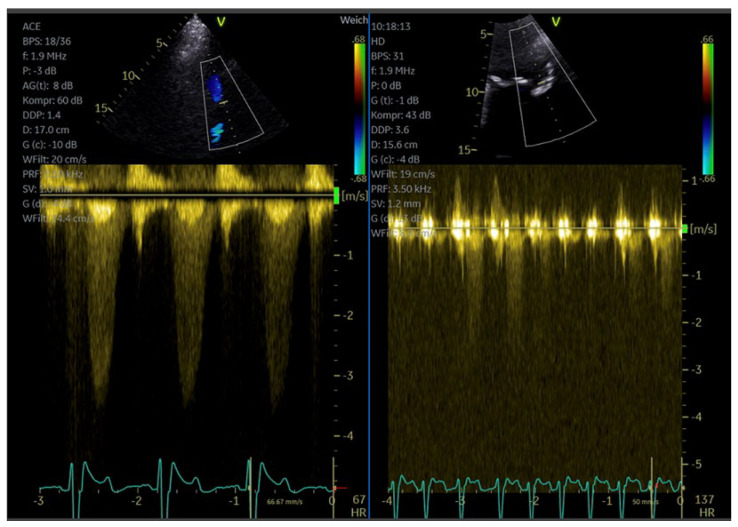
(Left) Contrast-enhanced CW Doppler recording of a patient with severe aortic valve stenosis, (right) unenhanced CW Doppler recording, Doppler envelops not closed, measurement of maximum velocity and velocity–time integral not valid.
